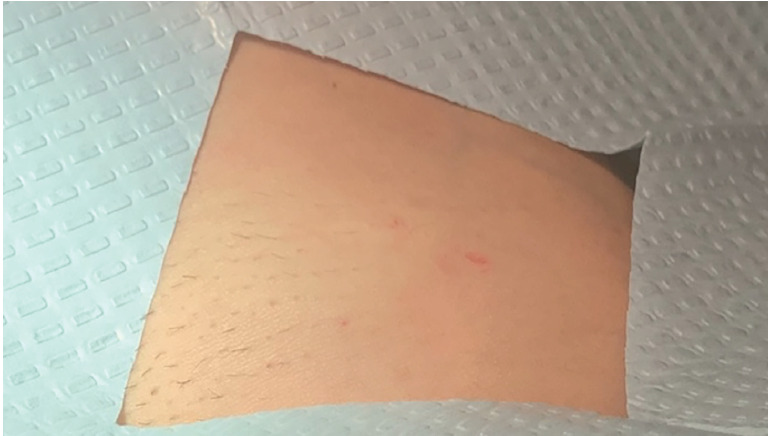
Pre-procedure image of right inguinal region demonstrating no clear puncture site for the foreign body visualized on computed tomography imaging.
